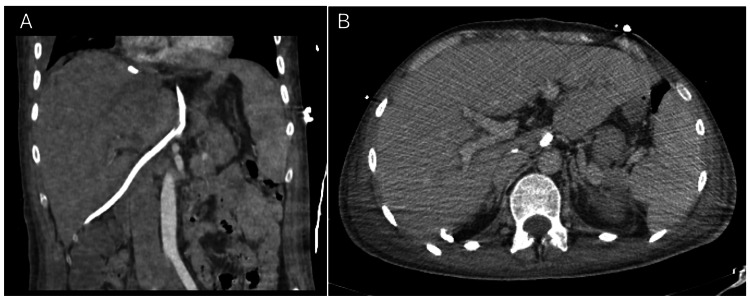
Contrast-enhanced CT scan of the upper abdomen. Contrast-enhanced CT scan of the upper abdomen in the arterial phase (A) and portal venous phase (B). The hepatic parenchyma appears heterogeneous, with areas of decreased attenuation compatible with hepatocellular necrosis (A). There is mild periportal oedema, with no evidence of a focal mass or biliary dilatation (B). The hepatic vasculature is patent. No ascites or focal collections are seen.
